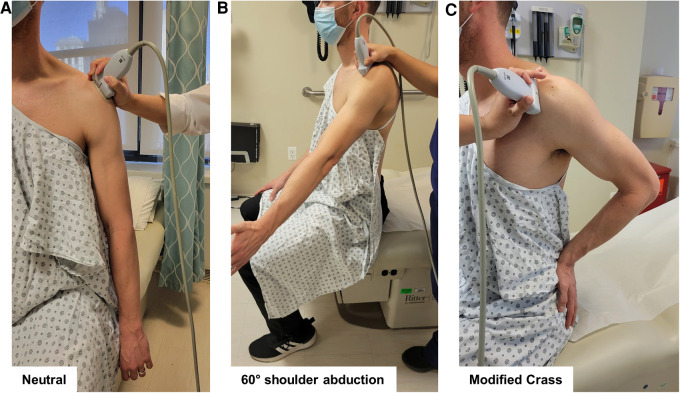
Participant positioning and transducer placement for shoulder ultrasound (US) evaluations. To measure acromioclavicular distance (AHD), each arm was examined in two positions: (A) in neutral position with the forearm pronated, resting on the ipsilateral thigh, and (B) at 60° active shoulder abduction in the coronal plane, as verified by goniometry. The US transducer is positioned in the coronal plane, parallel to the longitudinal axis of the humerus. To measure AGT distance, each arm was examined in neutral position with the forearm pronated, as described for AHD (A). To measure SST, SASDB, and SASDB fluid thickness, the participant was positioned with the palm of the examined arm resting on the posterior iliac crest and the elbow directed posteriorly (modified Crass position; (C). US transducer placement depicts positioning to obtain long axis views of the SST, SASDB, and SASDB fluid.
